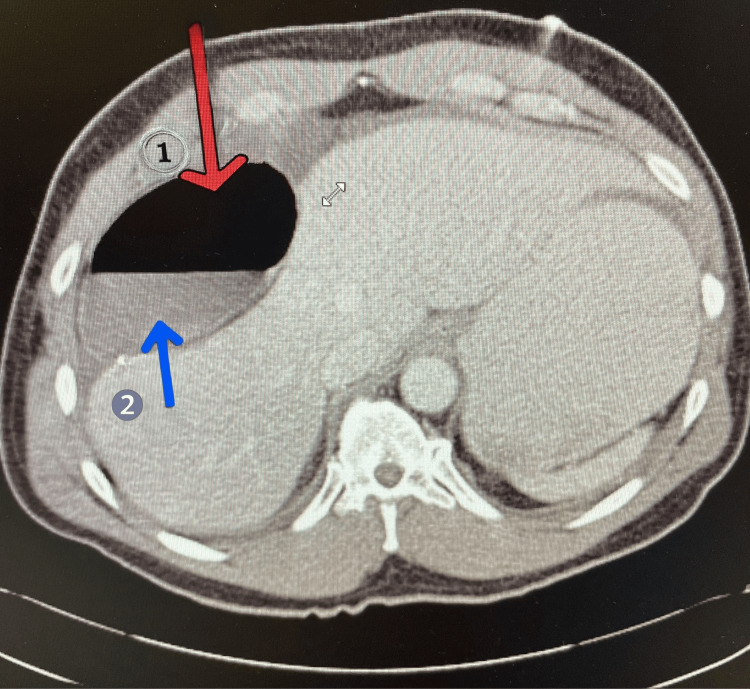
Repeat CT of the abdomen and pelvis in axial view. There is a well-defined, predominantly fluid-attenuation structure in the right upper quadrant, anterior to the liver, containing both hypodense (fluid) and hyperdense (air) components. The portion marked with the red arrow demonstrates a large intraluminal gas collection, suggesting a segment of bowel or gas within a cystic structure. The portion marked with the blue arrow shows dependent fluid, consistent with a fluid-fluid level within the same structure. There is surrounding free fluid within the peritoneal cavity. No obvious evidence of pneumoperitoneum or portal venous gas is identified. The liver, spleen, pancreas, and kidneys appear unremarkable in this section.
